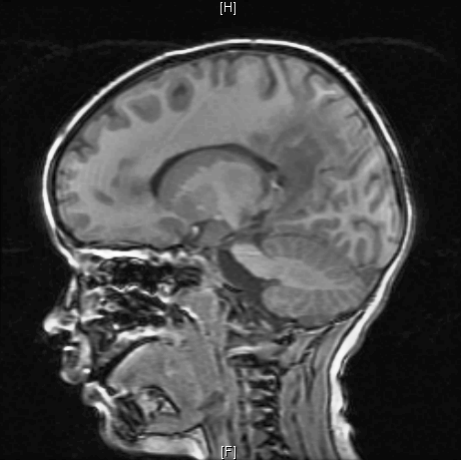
Magnetic resonance imaging of the brain showing bilateral symmetric demyelination of various parts of the brain
